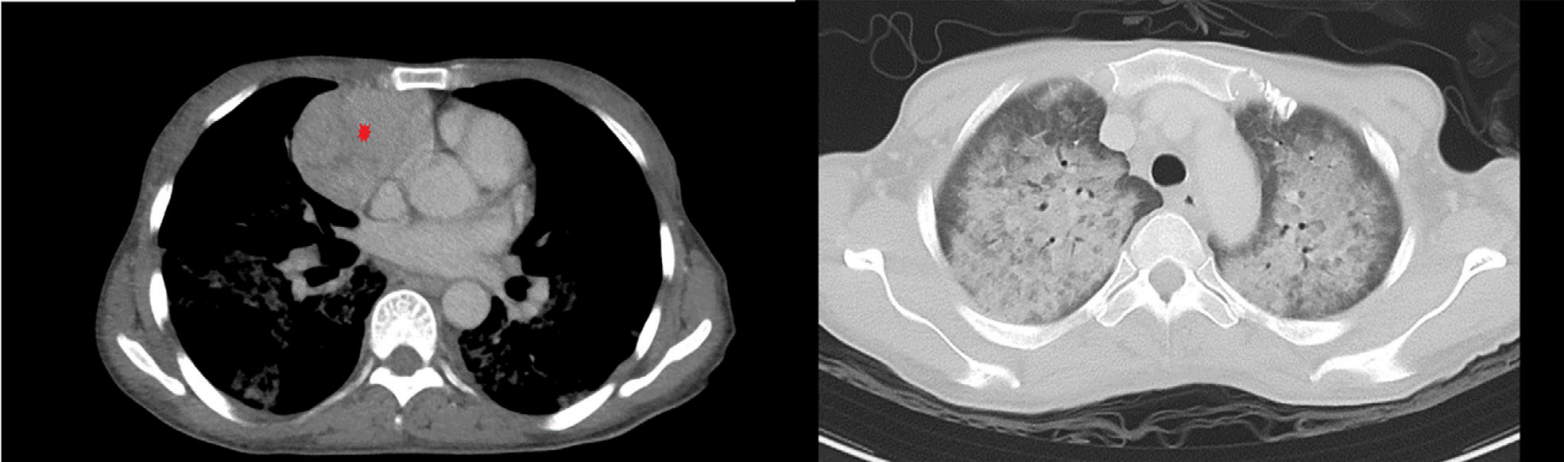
Contrast computed tomography of the thorax on day 7 of admission. Bilateral lungs confluent consolidations and ground‐glass densities with superimposed septal thickening are noted in all lobes, with upper zone predilection (right image). The anterior mediastinal soft tissue mass (marked with red star shown on the left image) measures 6.5 cm × 5.5 cm × 9.5 cm with hypo‐dense and hypo‐enhancing component. There are multiple enlarged mediastinal lymph nodes largest up to 1.2 cm at the left hilar region
